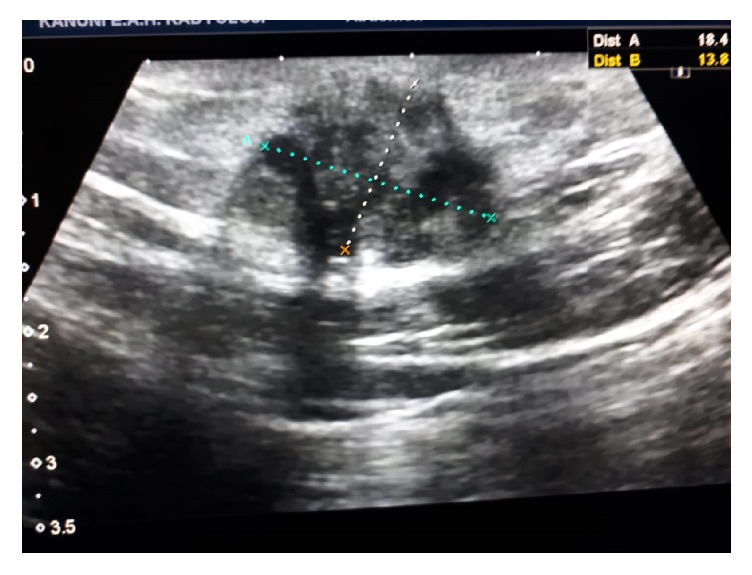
Abdominal USG shows an approximately 18 × 13 mm heterogeneous hypoechoic lesion with lobulated margins, which is localized between subcutaneous tissues and does not indicate vascularization in Doppler examination.
